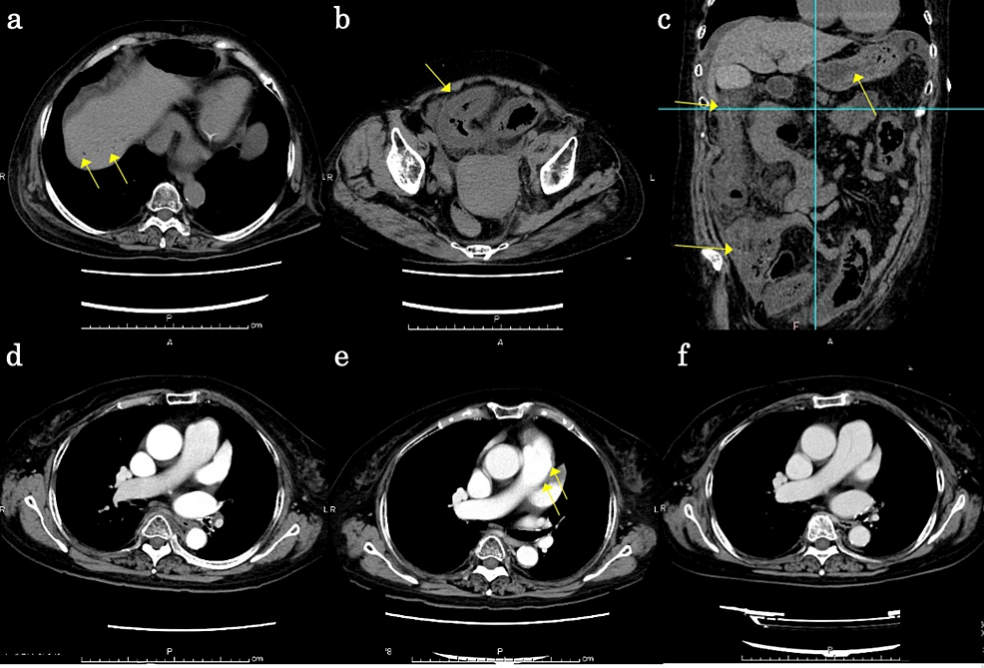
Abdominal and chest contrast-enhanced CT. CT scan showed ischemic colitis of the colon, free air in the liver portal vein (a), thickening of the gut wall in the transverse plane (b), and thickening of the gut wall in the coronal plane (c). CT scan of the chest showed complete enhancement of the left atrial appendage 10 days before the day of colitis (d), contrast enhancement defect on the day of colitis (e), and recovered enhancement of the left atrial appendage 10 months after the day of colitis (f).
